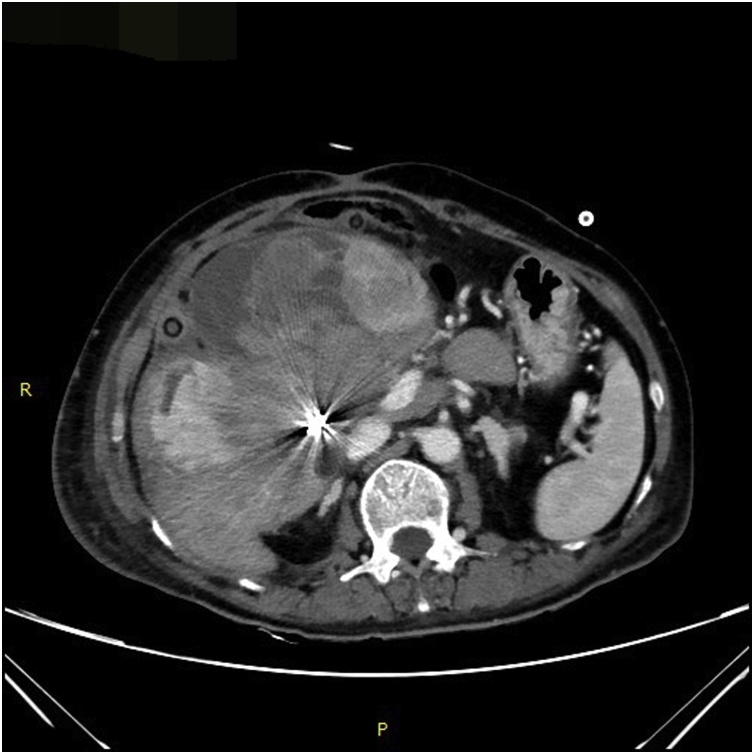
CT scan showing atrophy of liver, dilatation of bile duct, compression and displacement of hepatic portal system.
